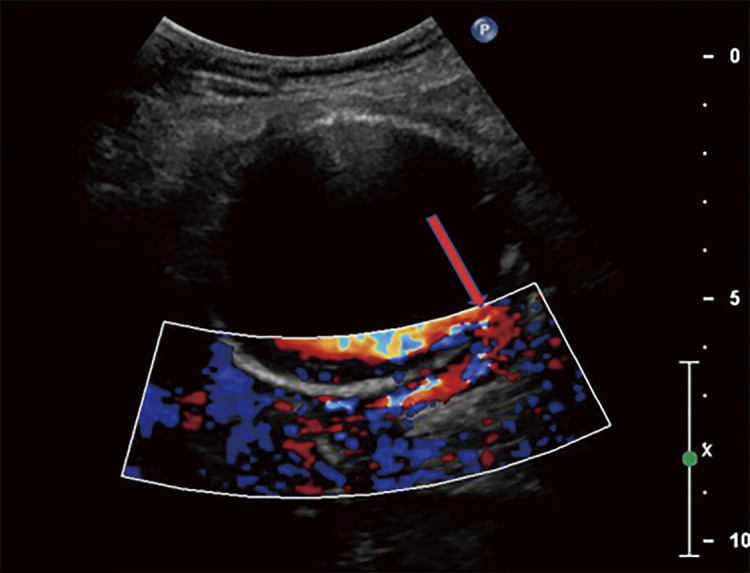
Abdominal ultrasonography revealing a right CIAA compressing the right CIV. Depicted are the absence of an intravenous thrombus and the presence of a 64 × 59 mm right CIAA, with shunt flow to the adjacent right CIV, where the proximal aspect is dilated (arrow). CIAA, common iliac artery aneurysm; CIV, common iliac vein
